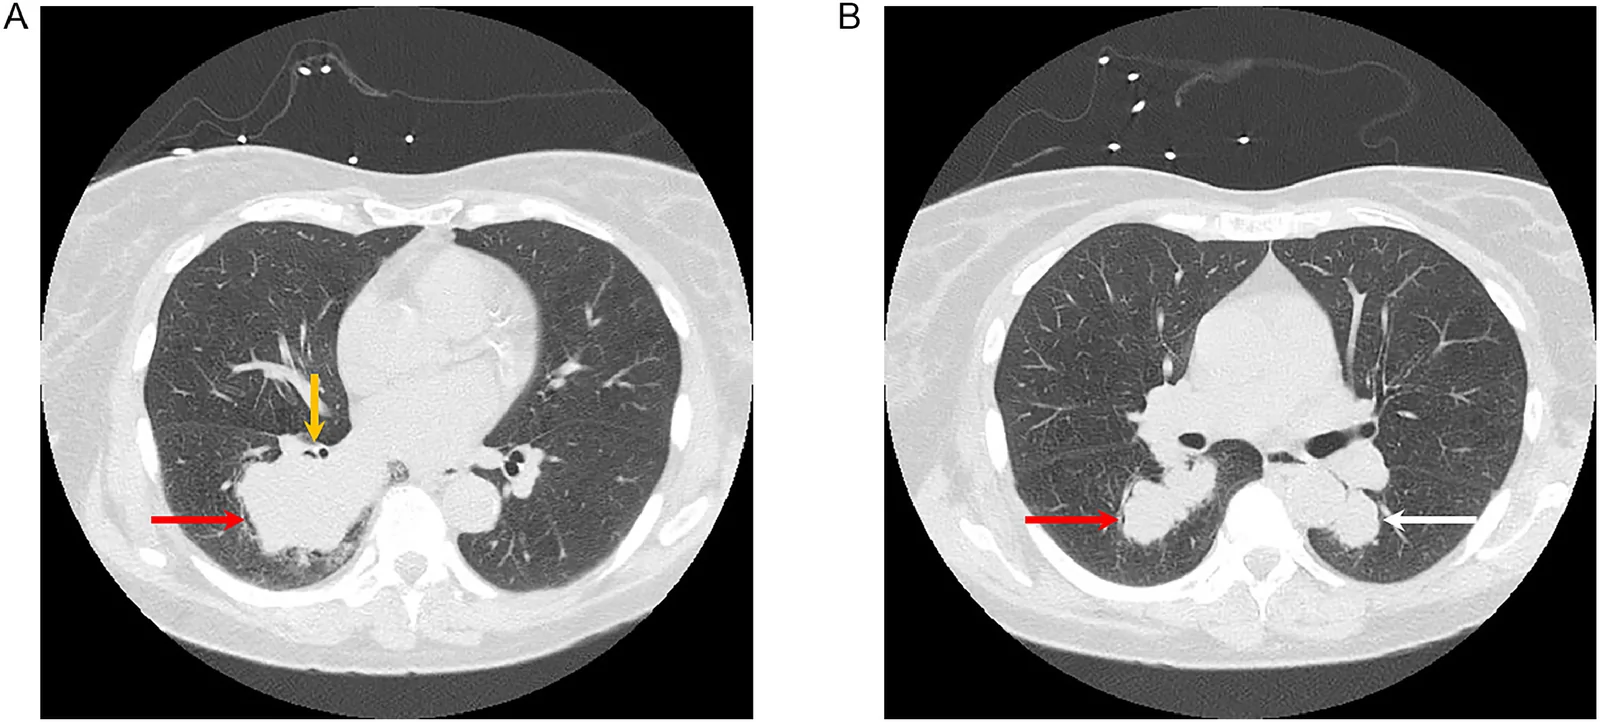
(A) Axial chest CT image demonstrates a large right lower lobe mass (red arrow), abutting/involving the bronchovascular bundle with partial effacement of a segmental bronchus (orange arrow). (B) Axial chest CT image (inferior to A) demonstrates a left lower lobe mass (white arrow) adjacent to the descending thoracic aorta, in addition to the right lower lobe mass (red arrow).
